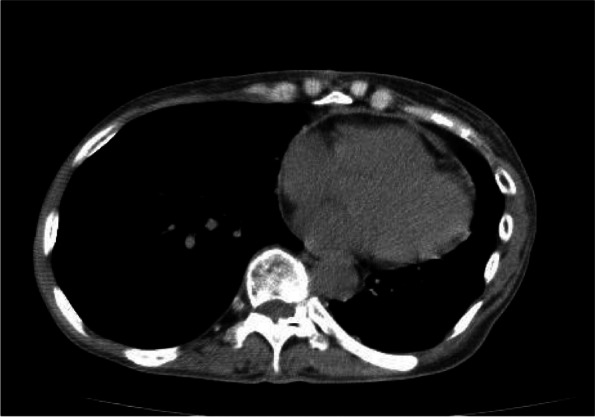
Chest CT 3 months after surgery. The patient had no recurrence of pericarditis at 3 months postoperatively
